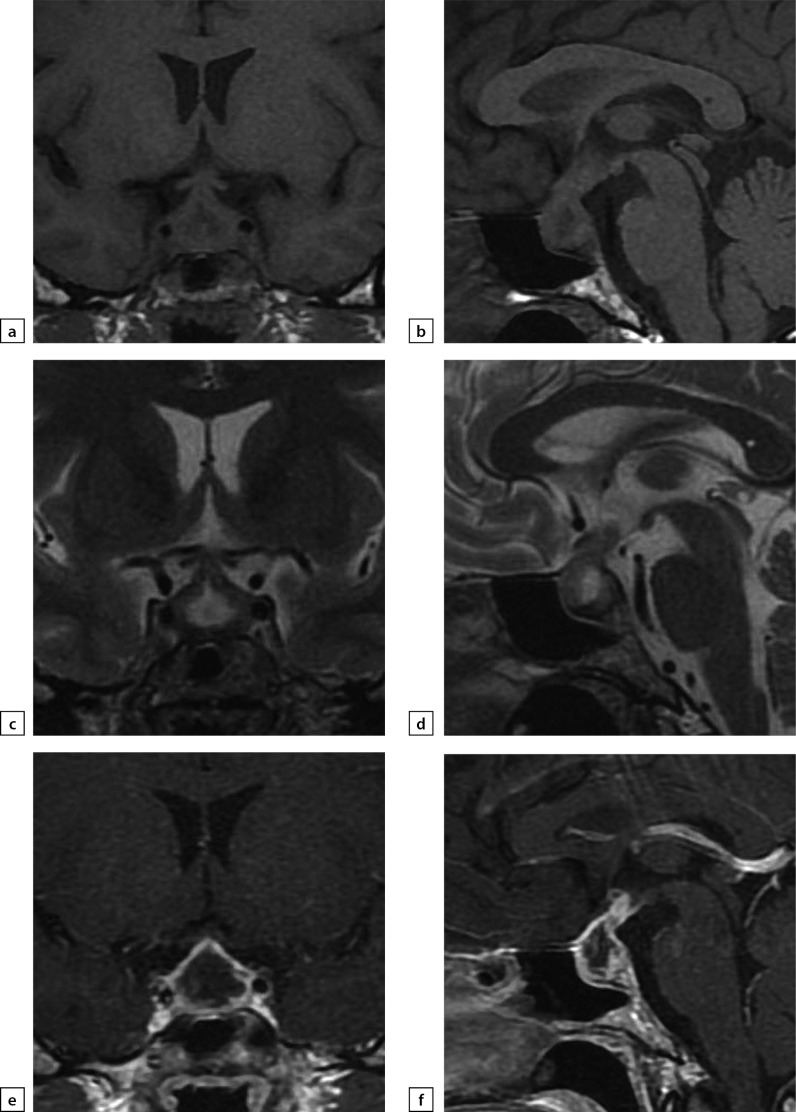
Figure 4. Female patient E (38)Lymphocytic hypophysitis. Substantial headaches, menstrual disorder, face numbness on the right-hand side. A MRI scan was conducted on 24 June 2019: a) T1WI, coronal slice; b) T1WI, sagittal slice; c) T2WI, coronal slice; d) T2WI, sagittal slice; e) T1WI, post-contrast coronal slice; f) T1WI, post-contrast sagittal slice. The scan found pituitary gland deformation with bulging upper end and inhomogeneous structure. At the centre, a liquid component with dimensions 5×7×5.5 mm was identified (infundibulohypophysitis). At pituitary gland periphery, intensive contrasting was observed. Pituitary stalk is deformed and evenly thickened to 4.5 mm; the stalk absorbs contrast agent intensively and unevenly. The typical signal from posterior pituitary is not identified. Suprasellar cistern is narrowed.
